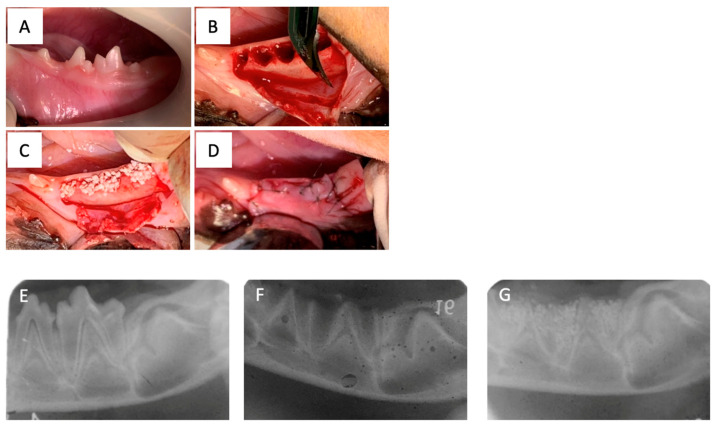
Treatment was performed as shown in (A–D) and confirmed by radiographs in (E,F). (A) Untreated left lateral mandibular molars (DP3 and DP4). (B) Post-extraction of the left mandibular molars (DP3 and DP4). (C) Bone graft material at the extraction site. (D) Closure of the grafted area of the bone grafting material. (E) Dental X-rays before treatment. (F) Dental X-rays at the extraction site. (G) Dental X-rays after treatment.
